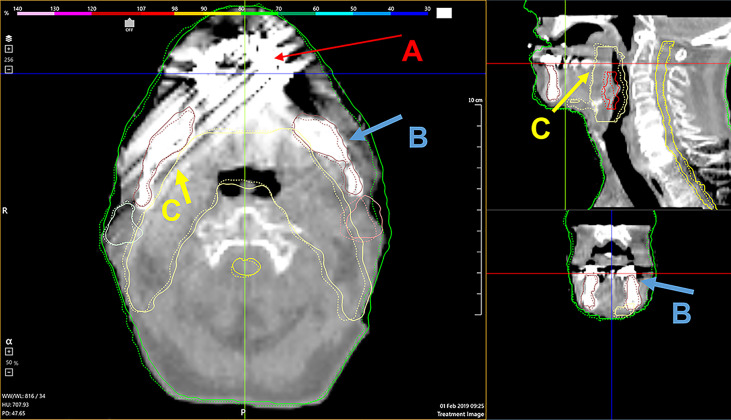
A demonstration of the deformable algorithm’s ability to account for severe CBCT dental implant artifacts [red arrows, (A)]. The blue (B) and yellow (C) arrows indicate the deformed mandible and PTV contour, respectively. Both were minimally affected by the streak artifacts.
